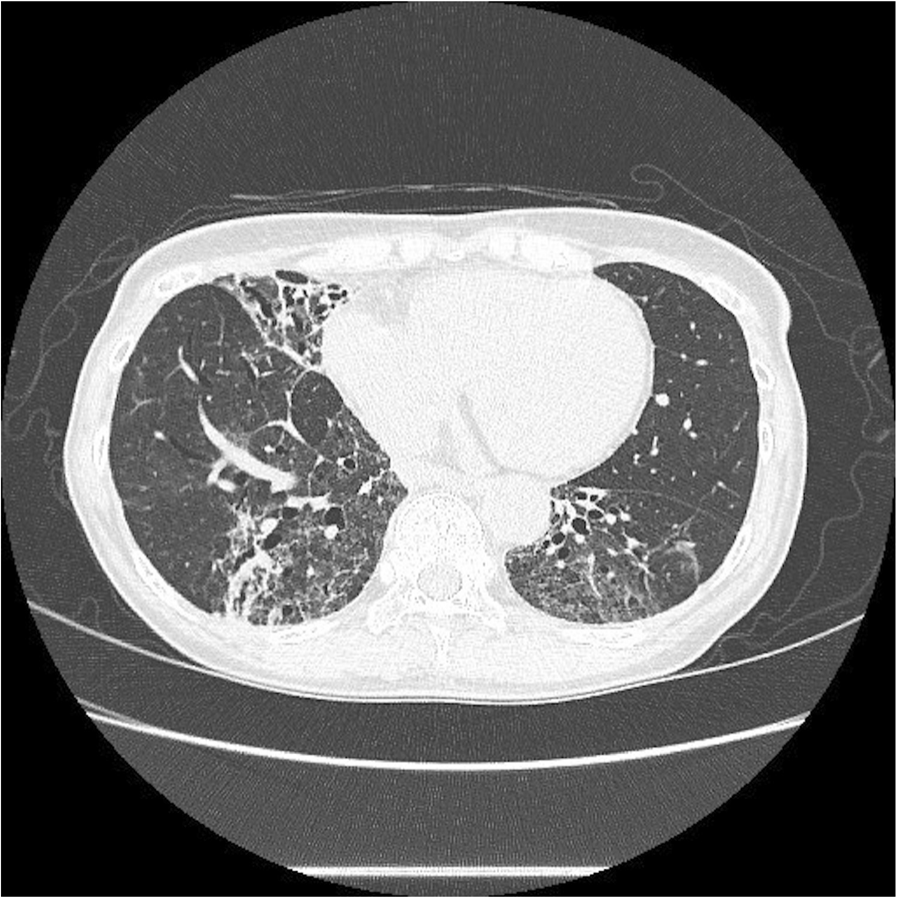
Chest CT image after the treatment
